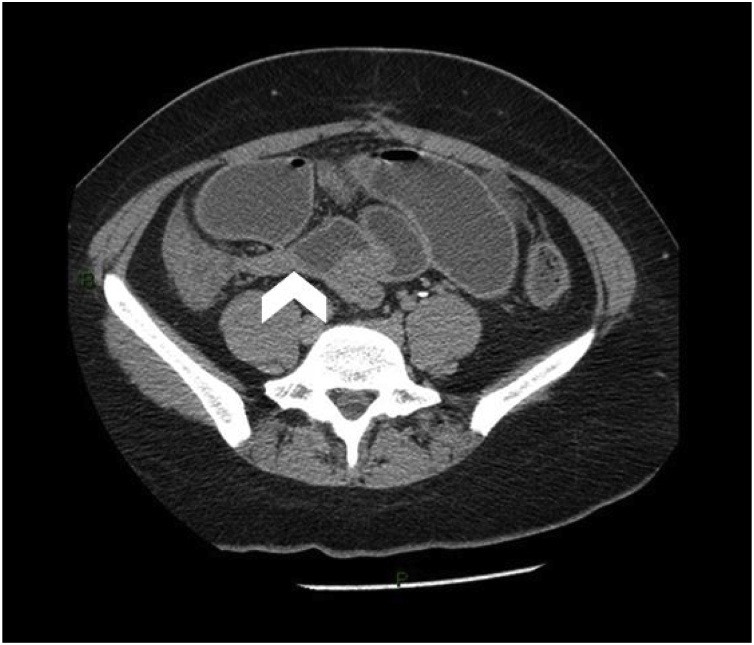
CT with IV contrast. Transition point at 5 cm from the ileocecal valve (white arrowhead).
